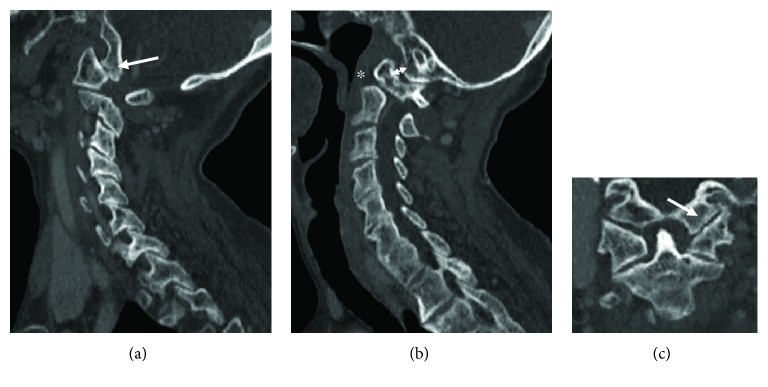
Left parasagittal (a) and coronal (c) CT scan images show a right nondisplaced impaction occipital condyle fracture (arrow), classified as Anderson and Montesano type I and Tuli type 1. Right parasagittal CT image (b) shows an occipital condyle-C1 interval of 2.5 mm (double-headed arrow) and a 5 mm translation of C1-C2 (asterisk).
